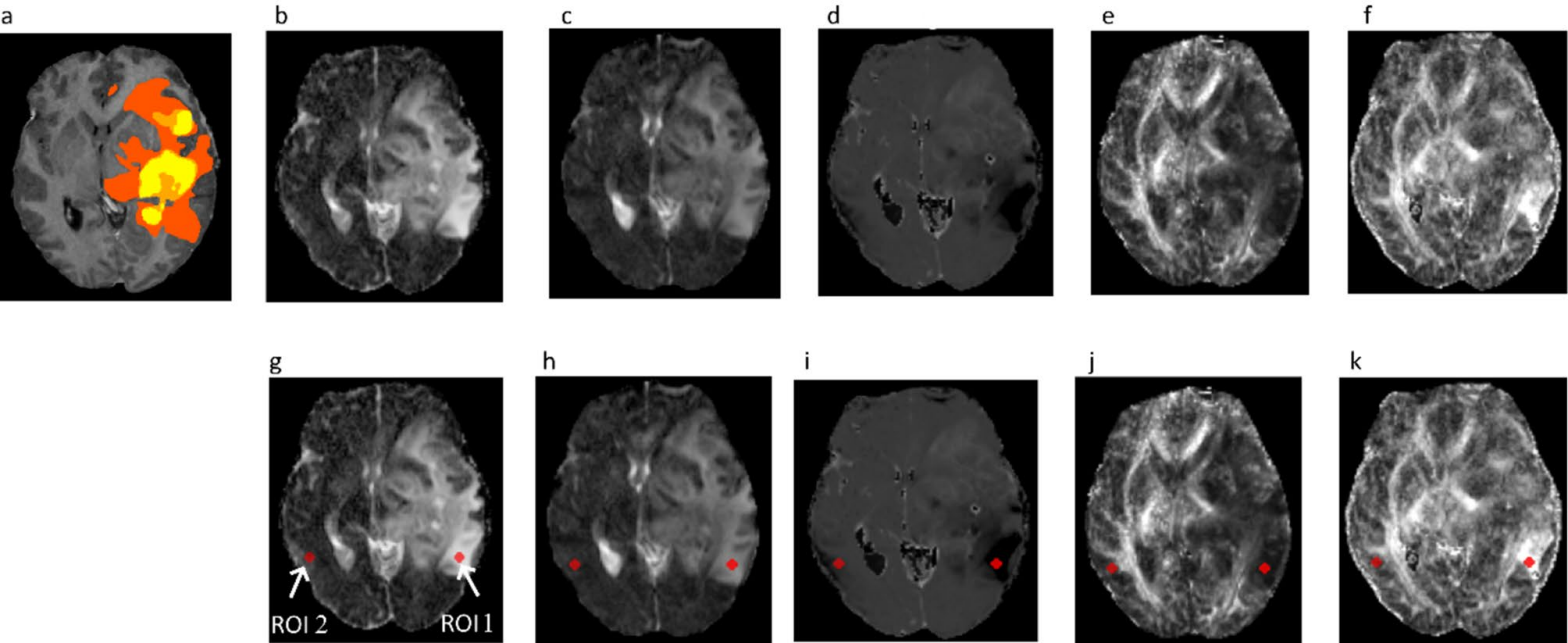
DTI analysis conducted on a typical HGG patient. (a) Shows the tumorous region superimposed on a T1-weighted image, (b,g) shows the FW map estimated using the FWE DTI model without and with ROIs, (c,h) shows MD map estimated using the standard DTI model without and with ROIs, (d,i) shows MD map estimated using FWE DTI model without and with ROIs, (e,j) shows the FA map estimated using the standard DTI model without and with ROIs, (f,k) shows the FA map estimated using FWE DTI model without and with ROIs. ROI 1 was placed in the edema region of the FW map, and ROI 2 was co-registered to the contralateral hemisphere using affine registration. All the above-mentioned operations were performed using FSL software.
